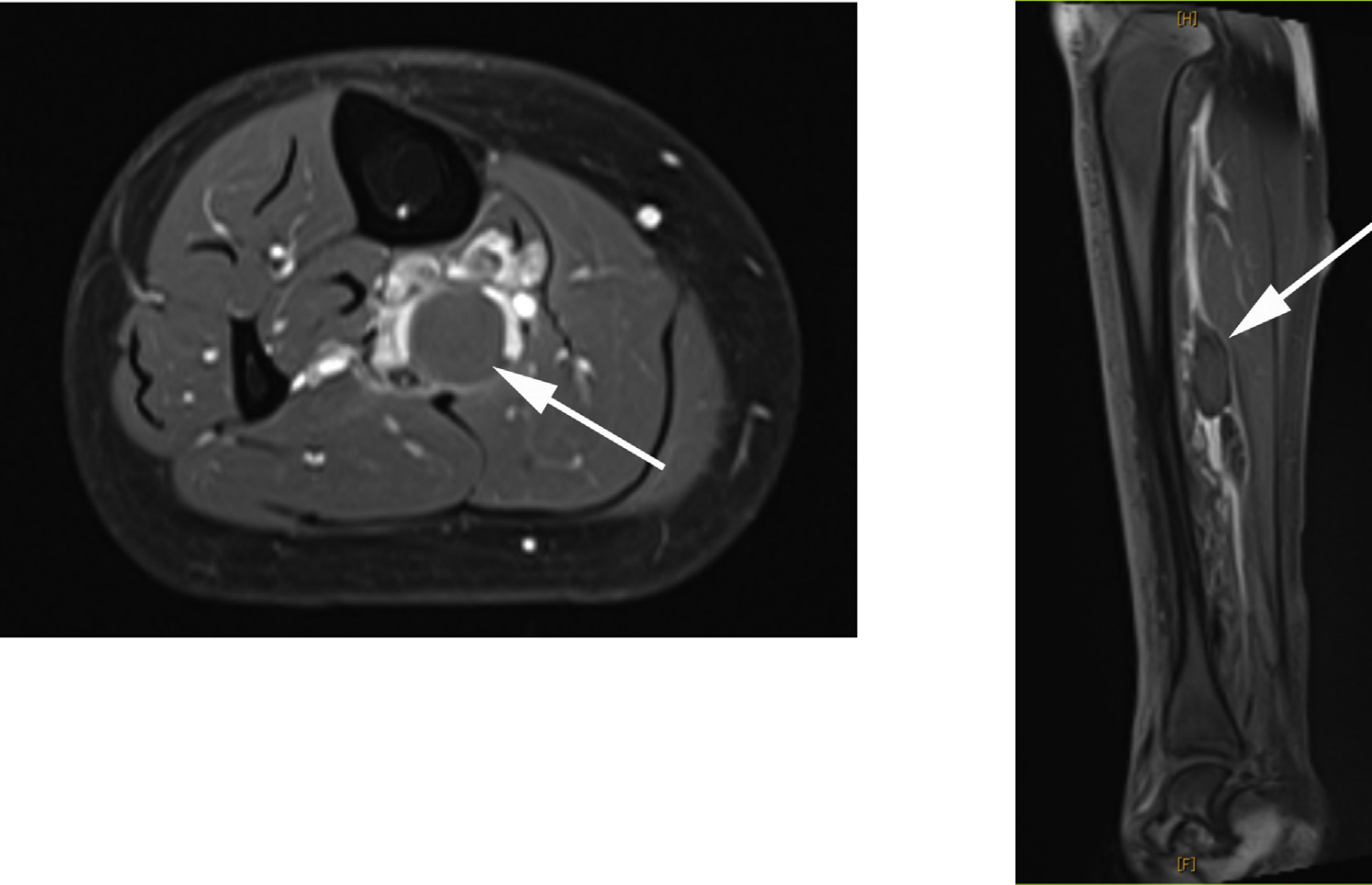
Left, Contrast-enhanced magnetic resonance image showing the posterior tibial vein aneurysm (*white arrow*) in axial view. Right, Three-dimensional computed tomography image showing the posterior tibial vein aneurysm (*white arrow*).
